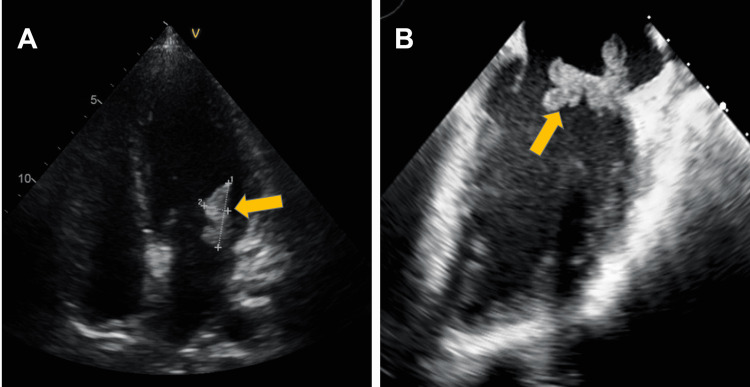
Transthoracic and transesophageal echocardiography (A) Preoperative transthoracic echocardiography demonstrates mobile vegetations on posterior mitral leaflets (arrow). (B) Transesophageal echocardiogram in where a large, mobile vegetation is observed, adhered to the posterior leaflet of the mitral valve.
